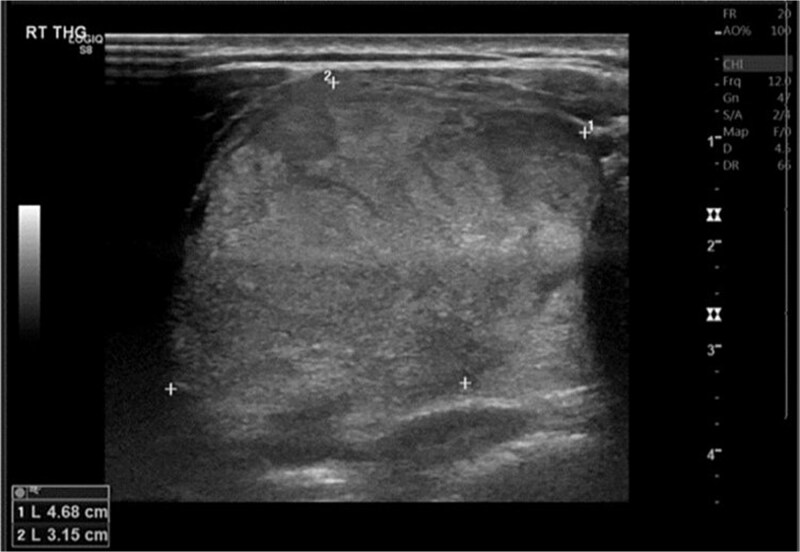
Thyroid ultrasonography reveals an enlarged right lobe of the thyroid.
